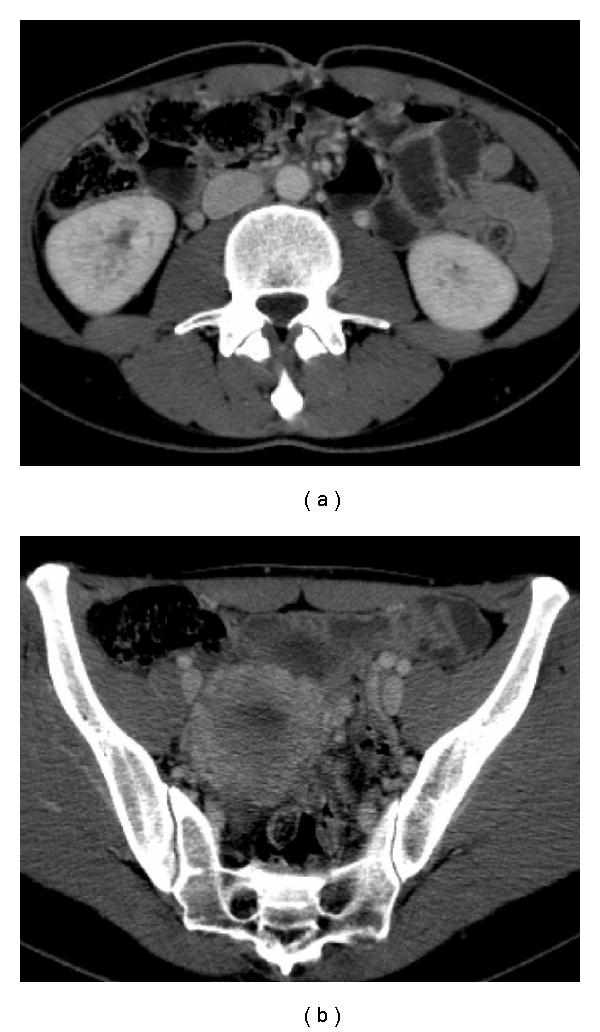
Retrospective evaluation of CT images showed a great dilation of the ovarian veins and of the periuterine venous plexus.
